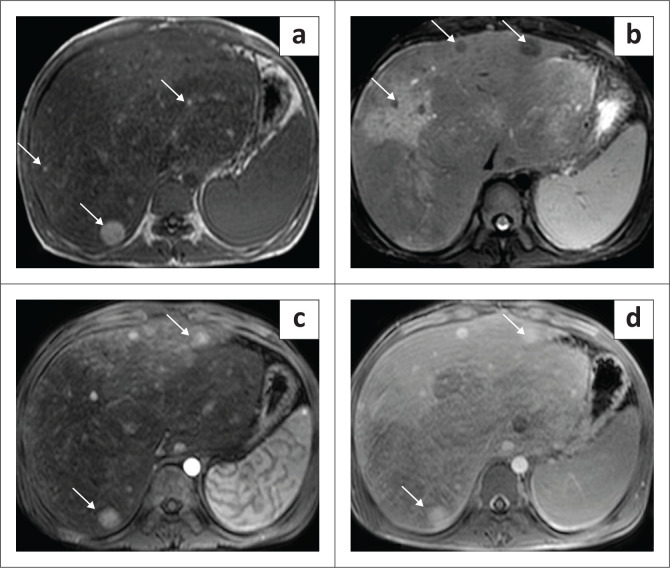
(a–d) MRI of multiple nodular regenerative hyperplasia in a child with hepatic venous outflow obstruction. Axial T1-weighted (T1W) (a) and T2-weighted (T2W) fat saturated (b) images of the upper abdomen showing multiple tiny lesions which are hyperintense and hypointense, respectively, involving both the lobes of liver. There is associated hepatomegaly. The post-contrast arterial phase image (c) shows intense contrast enhancement of all the lesions (arrows) which also demonstrate contrast retention on the portal venous phase (d).
